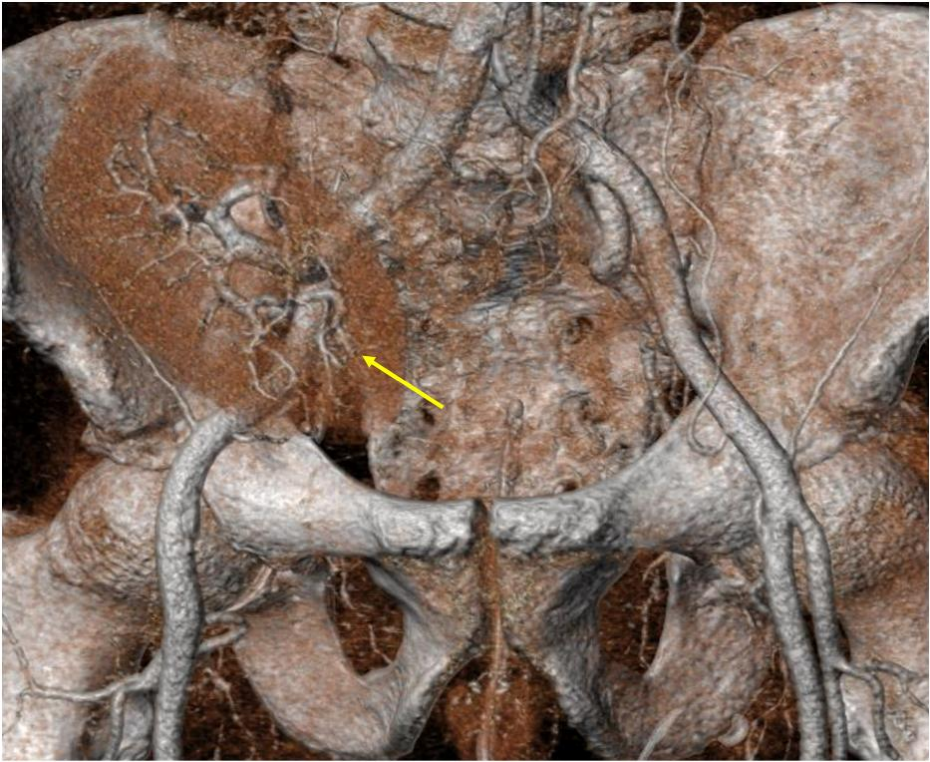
A computed tomography image demonstrating a heterotopic kidney transplant with a vascular pedicle coming off the right iliac artery (arrow) and a suitable left femoral approach for a transcatheter heart valve implantation.
